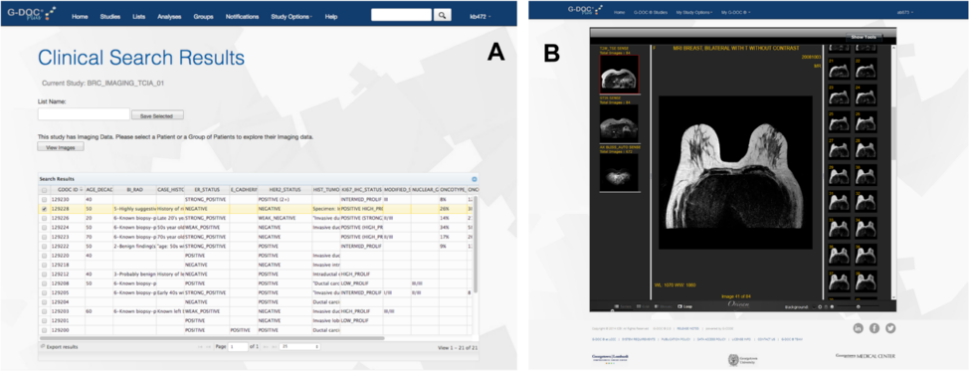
a Selection of medical images based on clinical data of patient(s). b Screen shot of the medical MRI imaging module in G-DOC *Plus*
